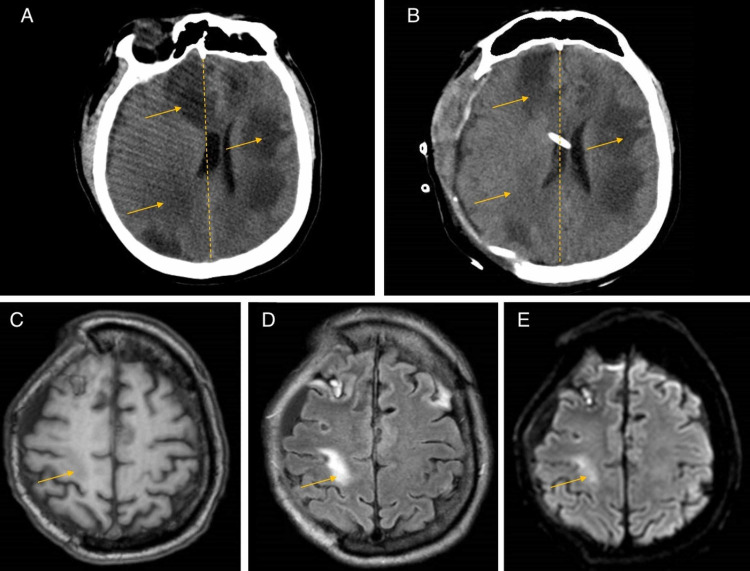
Peri-operative CT brain imaging and three-month postoperative MR brain imaging (A) Pre-operative CT head image demonstrating mass effect and midline shift more significant on the right side. (B) Postoperative CT head image after right decompressive hemicraniectomy. (C) Three-month postoperative T1-weighted post-contrast MR image demonstrating marked improvement of lesions. (D) Three-month postoperative T2-weighted-fluid-attenuated inversion recovery (T2-FLAIR) image further demonstrating an improvement of multifocal sites of confluent white matter, with the continuing resolution of vasogenic edema. (E) Three-month postoperative diffusion-weighted imaging (DWI) MR image demonstrating resolution of disease.
